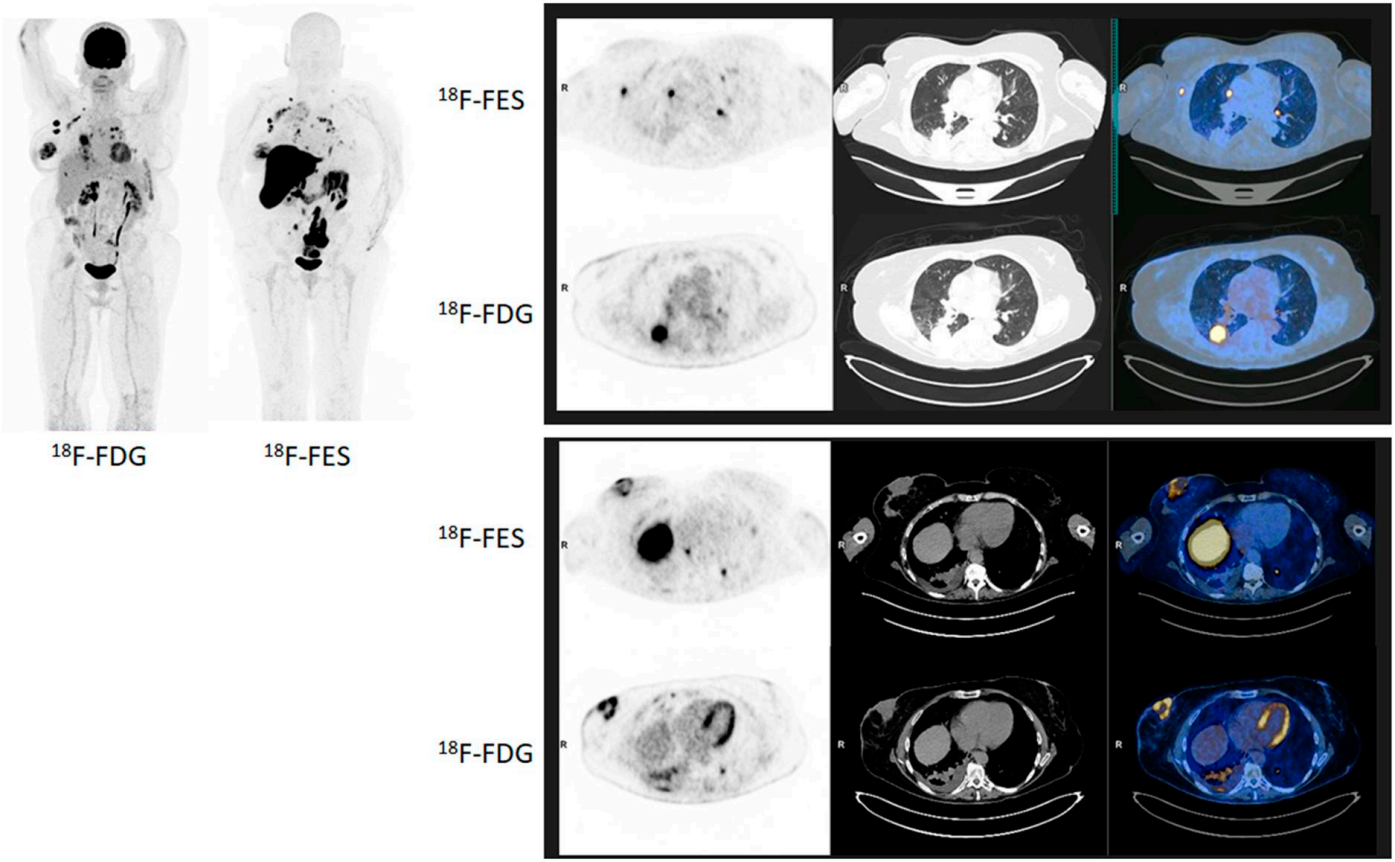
A 59-year-old woman presented with a right breast lesion after local trauma. Clinical examination revealed an ulcerated lesion involving the entire areola and bleeding on contact. Biopsy confirmed an invasive ductal carcinoma grade II, estrogen receptor positive (ER+), progesterone receptor negative (PR–), and HER2 negative (0). A staging [¹⁸F]FDG PET/CT demonstrated intense hypermetabolism of the right breast lesion, multiple hypermetabolic axillary lymph nodes, a large right lower lobe pulmonary mass, mediastino-hilar lymphadenopathies, multiple nodular lesions, and an osteolytic lesion in the left sacral wing. The lung lesion also shows intense uptake of [¹⁸F]FDG, although to a slightly lesser degree than the breast lesion. The pulmonary lesion was large and demonstrated intense [¹⁸F]FDG uptake, raising suspicion for a second primary malignancy rather than a metastasis. Given this metabolic pattern, a 16α-[¹⁸F]fluoro-17β-estradiol ([¹⁸F]FES) PET/CT was subsequently performed to assess estrogen receptor expression and further characterize potential metastatic disease. The scan revealed a dissociated uptake pattern, with no [¹⁸F]FES uptake in the right lower lobe pulmonary mass or mediastino-hilar lymphadenopathies, whereas the sacral and iliac bone lesions demonstrated FES uptake, consistent with ER-positive breast cancer metastases. CT-guided biopsy of the pulmonary lesion confirmed a primary bronchopulmonary adenocarcinoma, ALK-negative and ROS1-negative, PD-L1 20%, harboring BRAF V600E and PIK3CA mutations. These findings established the coexistence of 2 synchronous metastatic primaries: an ER-positive breast carcinoma and a BRAF-mutated lung adenocarcinoma. Targeted therapy with dabrafenib and trametinib was initiated for the metastatic lung adenocarcinoma, whereas hormonal therapy with an aromatase inhibitor was continued for the breast cancer. At the 3-month follow-up, CT imaging showed a partial response, with regression of the pulmonary lesion, mediastinal lymph nodes, and carcinomatous lymphangitis, whereas the right breast mass remained stable. Clinical tolerance to therapy was good. Double primary malignancies involving the breast and lung are well-documented in the medical literature.^1^ The diagnostic value of [¹⁸F]FDG PET/CT in the staging and management of breast cancer is well established,^2^ as is that of [¹⁸F]FES PET/CT for assessing estrogen receptor expression.^3–7^ However, reports describing synchronous breast and lung primaries evaluated with both radiotracers remain extremely rare. To our knowledge, only one case, reported by Lee et al,^8^ described a similar presentation but without available [¹⁸F]FDG imaging. In conclusion, [¹⁸F]FES PET/CT played a pivotal role in differentiating synchronous primary malignancies from metastatic dissemination, enabling accurate molecular characterization and optimization of therapeutic management in this complex oncologic setting.
